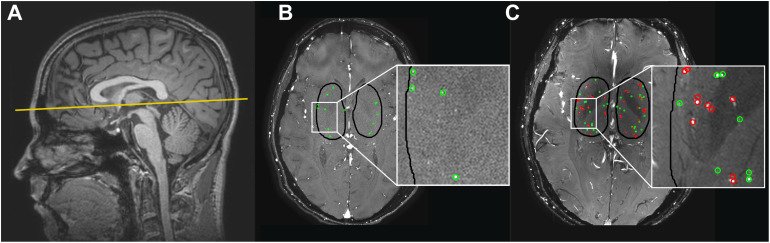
(A) The 2D phase contrast slice in the basal ganglia (BG) is located at the bottom of the corpus callosum (solid line). (B,C) Cerebral perforating arteries detected in the BG with 3 tesla (T) MRI (B) and 7T MRI (C) and included in the analysis are circled in green. Cerebral perforating arteries excluded from analysis due to a non-perpendicular orientation or close proximity to other perforating arteries (<1.2 mm) are circled in red. Note that for this subject all detected vessels at 3T were sufficient for inclusion. Also note the inhomogeneity in the background suppression at 7T (darker central area), which is induced by the well-known inhomogeneity of the radiofrequency B_1_^+^ transmit field (resulting in spatially varying flip angle) at 7T MRI (Van De Moortele et al., 2005).
